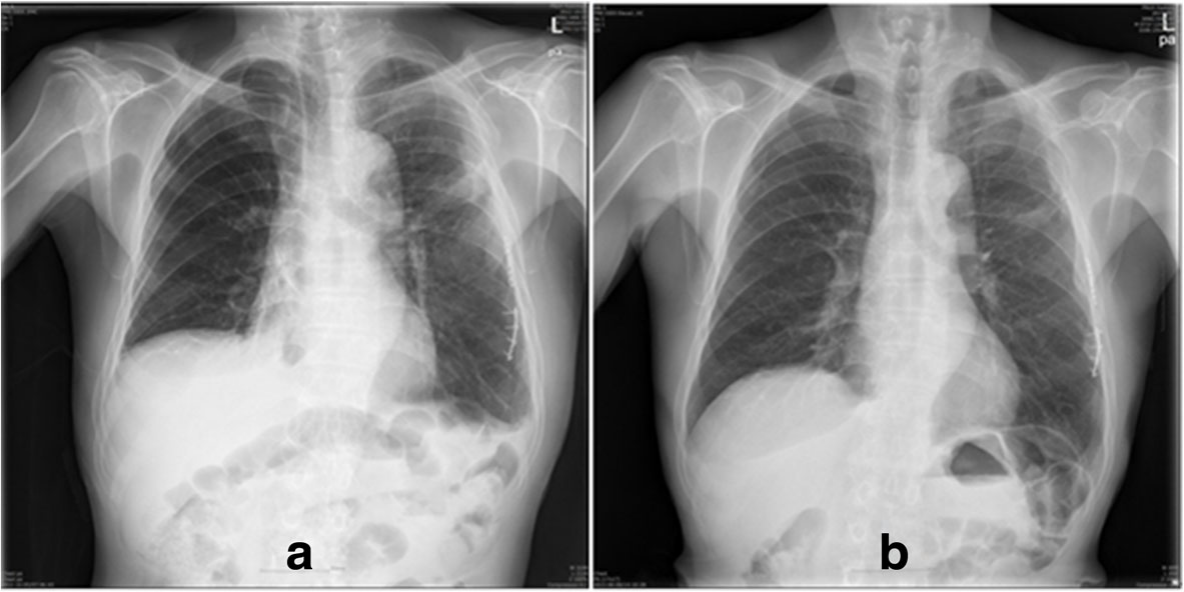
a Chest X-ray at discharge day. b Chest X-ray at 1 year after treatment
